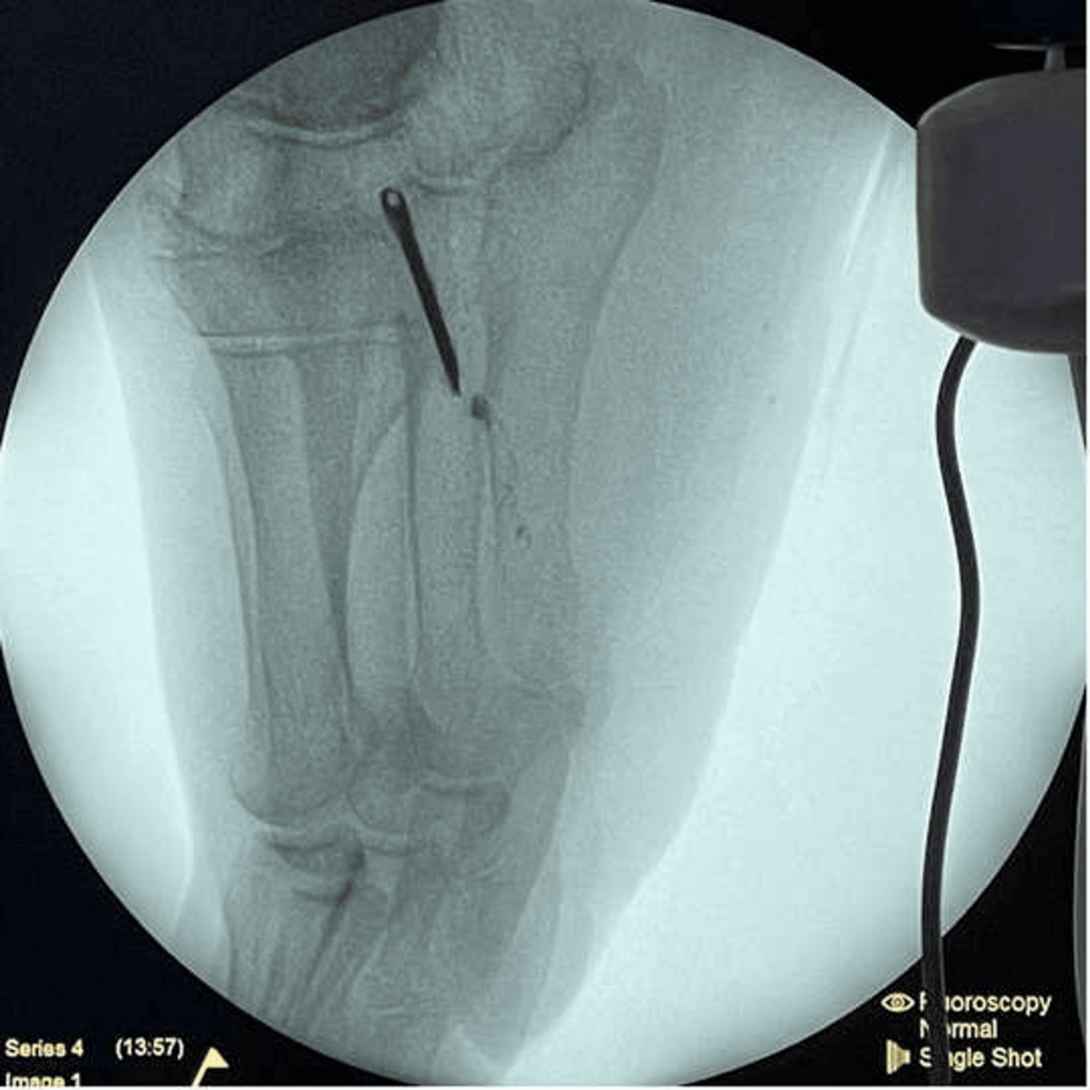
Final radiograph with the residual foreign body.
